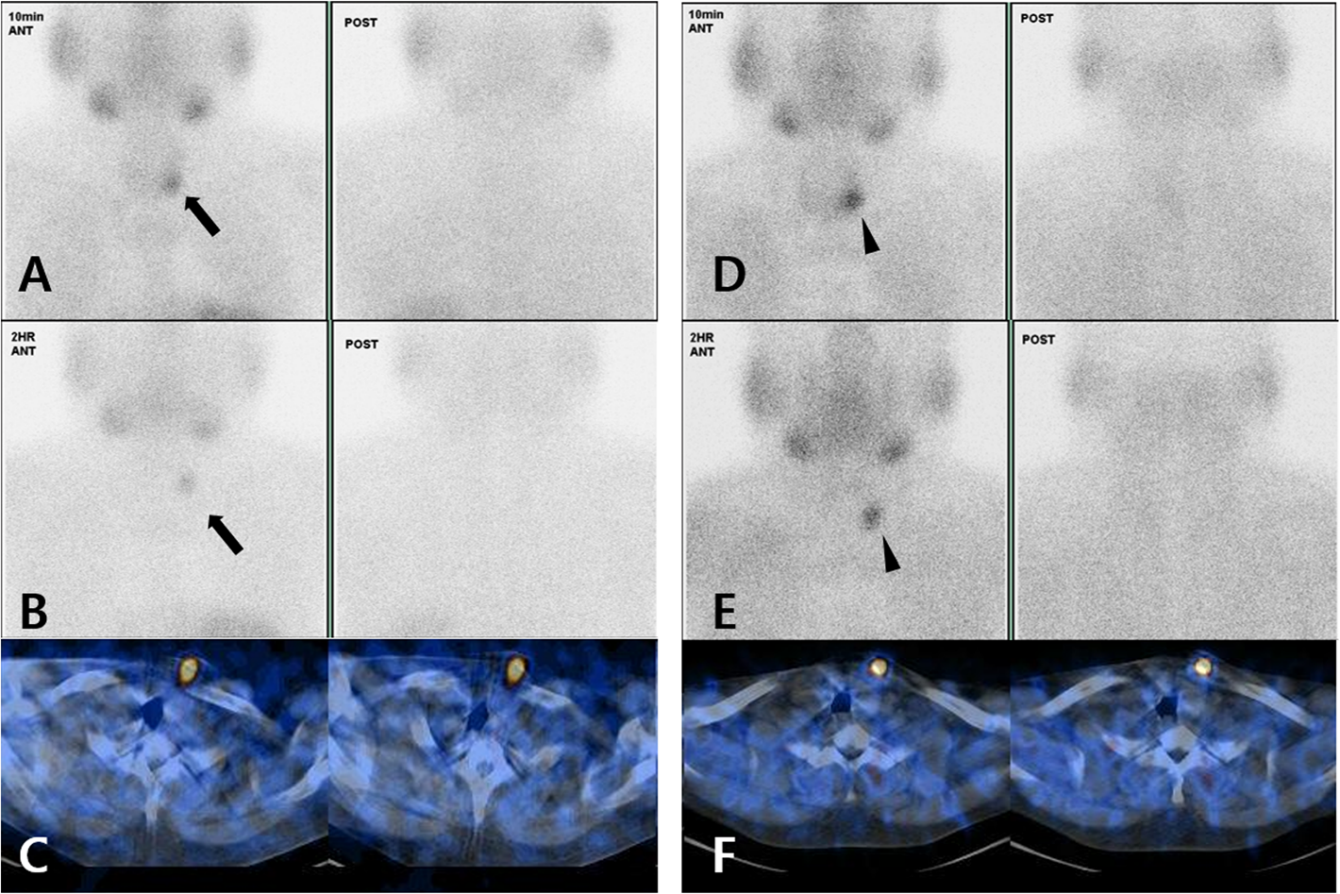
Tc-99 m MIBI dual-phase parathyroid scintigraphy. a b cFocal increased uptake in clavicular head area of left sternocleidomastoid muscle (black arrow). Parathyroid Scintigraphy performed 3 years after total parathyroidectomy with auto-transplantation; (A) Early and wash-out images acquired at 10 min post injection; (b) Delayed and wash-out images acquired at 120 min post injection; (c) Axial fused images acquired at 120 min post injection; (d) (e) (f) A hyperfunctioning auto-transplanted parathyroid tissue with increased uptake was identified compared to previous scintigraphy (black arrowhead). Parathyroid Scintigraphy performed 8 years after total parathyroidectomy with auto-transplantation; (d) Early and wash-out images acquired at 10 min post injection; (e) Delayed and wash-out images acquired at 120 min post injection; (f) Axial fused images acquired at 120 min post injection
